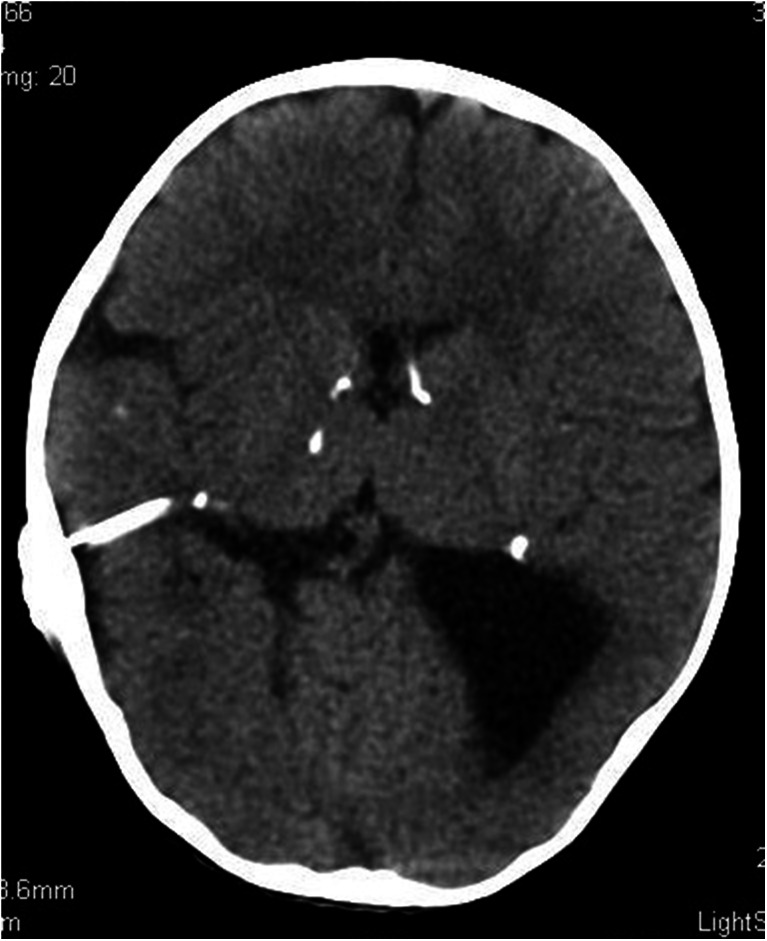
The scan was performed 7 months following ventriculoperitoneal shunting. Marked supratentorial hydrocephaly regression. Mildly distended occipital and temporal horns of the left lateral ventricle. Nonenhanced CT scan
